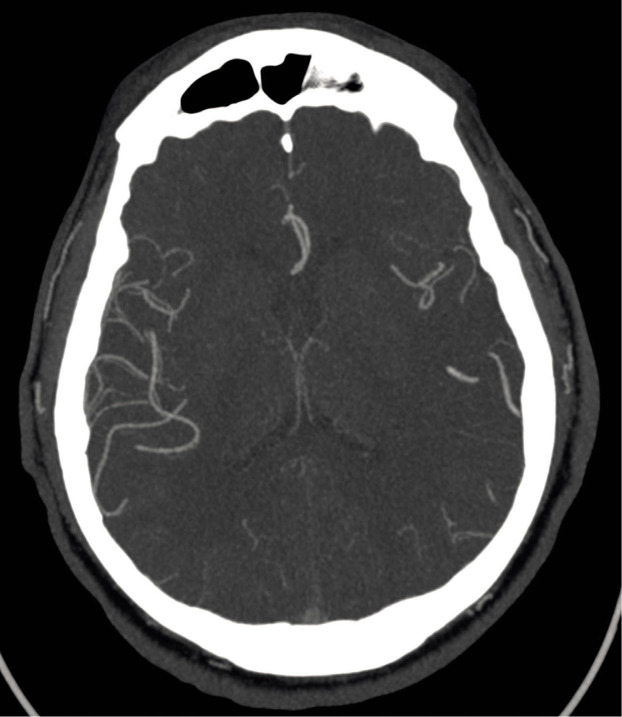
Head computed tomography scan showing a cerebral infarction in the left middle cerebral artery territory with occlusion and impaired distal blood flow
